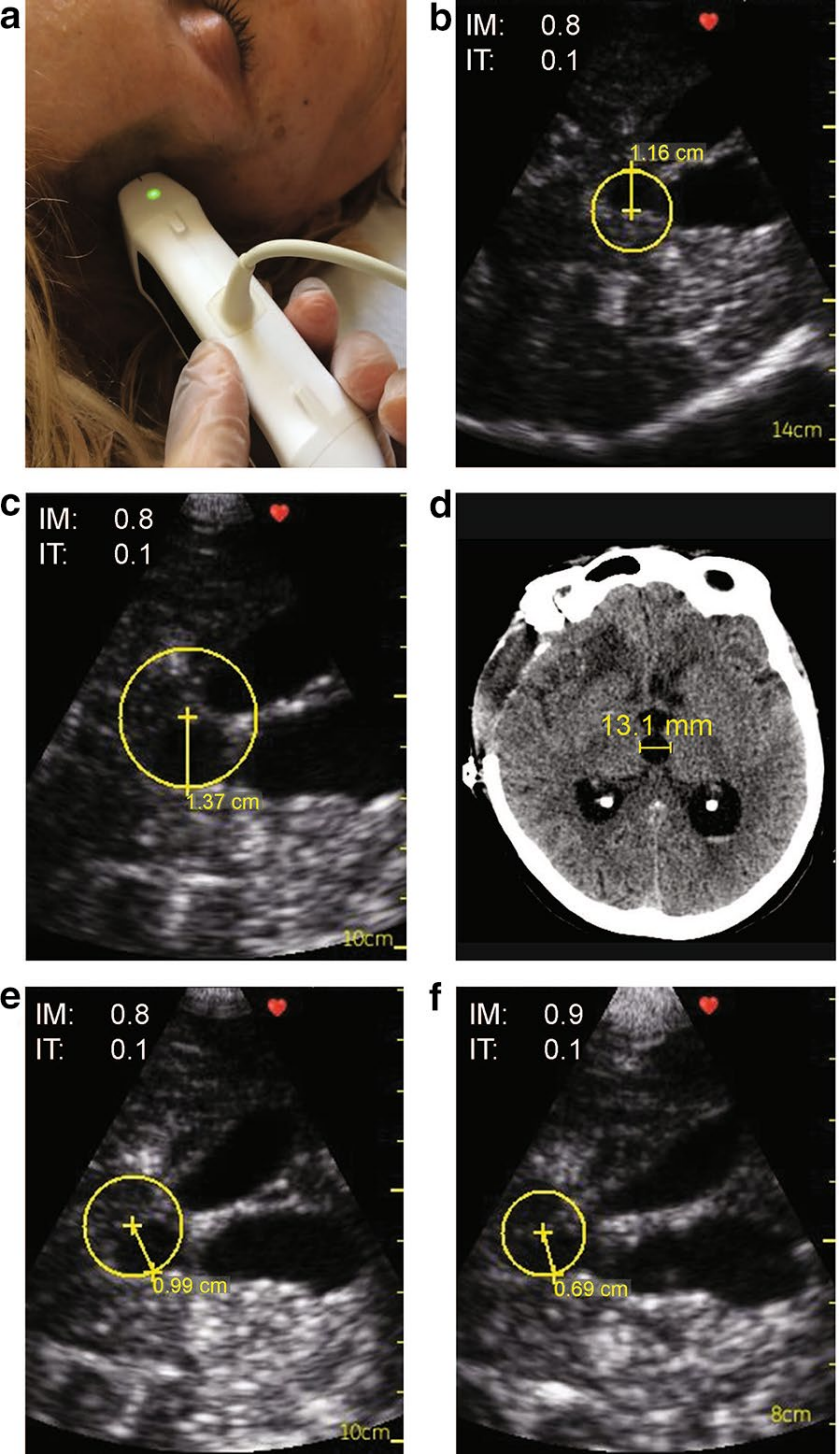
a TCS using hand-held pocket ultrasound device (GE Vingmed Ultrasound AS, Horten, Norway). b Prior to EVD closure, TCS showed a measurement of the 3rd ventricle at around 1.16 cm. c On the third day, TCS showed a dilated 3rd ventricle measuring 1.37 cm. d CT scan showed a dilated 3rd ventricle measuring 13.1 mm. e Subsequently following EVD re-opening, on the next day using TCS the 3rd ventricle dimension was reduced to 0.99 cm. f The following day it went down to 0.69 cm
